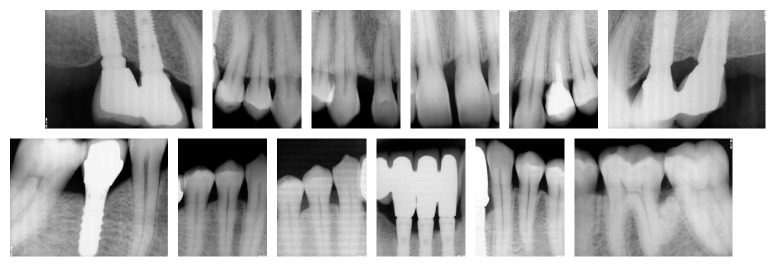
Radiographic evaluation 10 years after prosthesis insertion.
